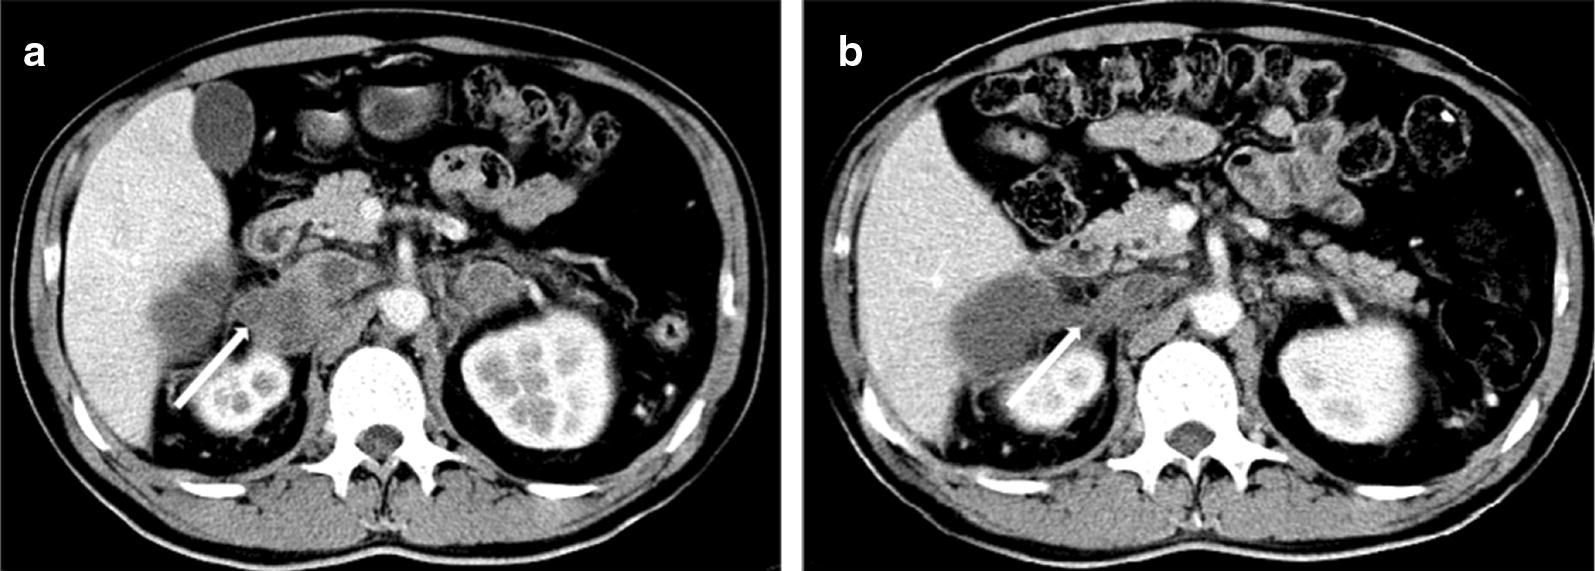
Representative CT scanning result of one patient with acquired MET amplification who responded to crizotinib monotherapy. a Before treatment; b partial response was detected after 1 month of treatment
